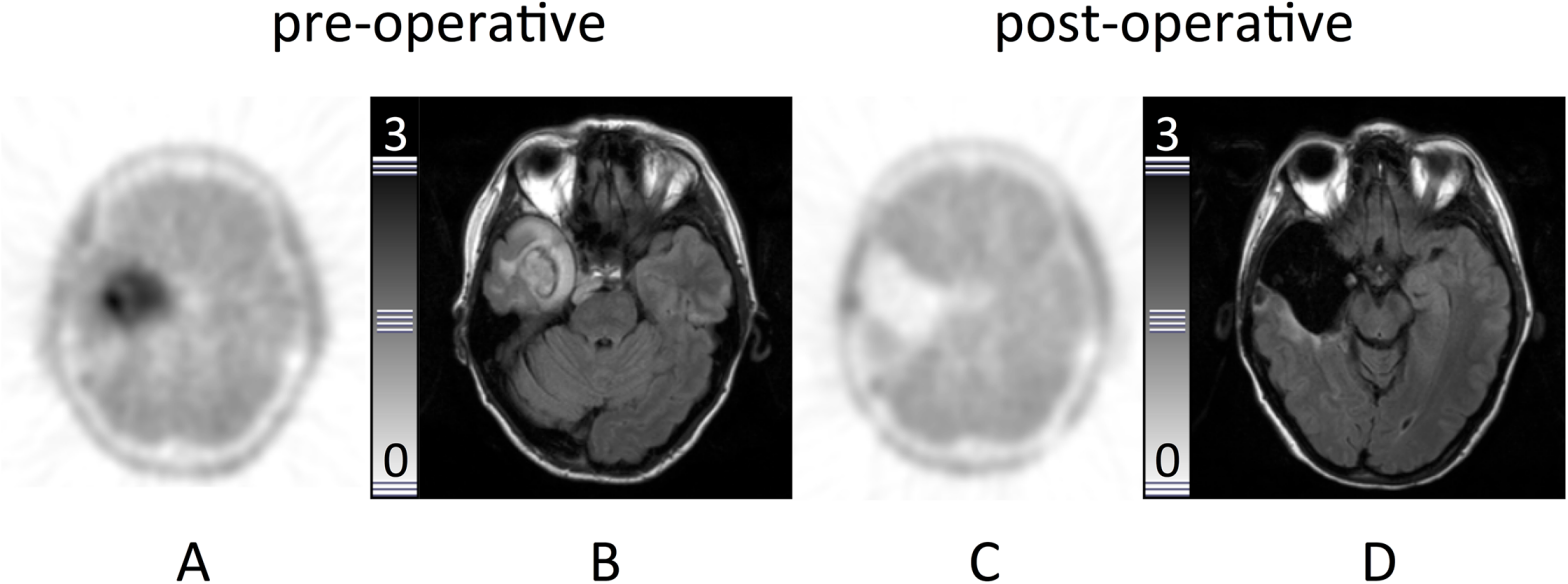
Example of a complete resection. Patient #2. Transaxial slices. A: Intense focal [^18^F]FET uptake in the right temporal lobe. B: MRI (FLAIR-sequence) with diffuse hyperintensity in the same region. C: [^18^F]FET-PET (48h after resection) and D: MRI (24 h after resection, FLAIR) with no signs of residual tumor tissue.
